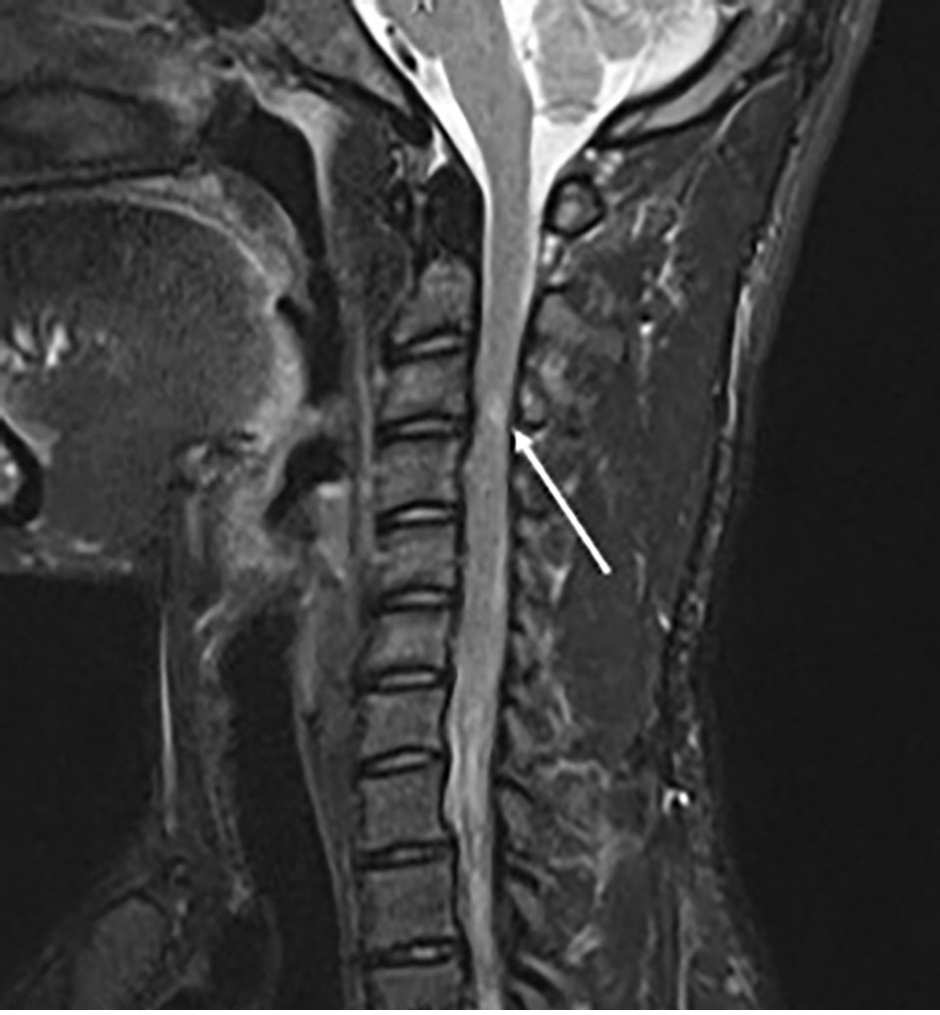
Magnetic resonance imaging with arrow demonstrating cervical spinal cord edema at the site of congenital spinal canal narrowing of 11 millimeters.
